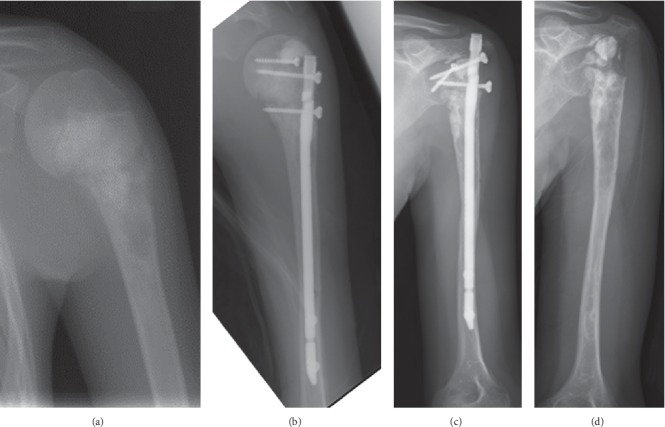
Case #6: 12-year-old female with osteosarcoma of the left proximal humerus. (a) Preoperative X-ray. (b) Immediately after treatment with osteoarticular extracorporeal irradiated autograft. (c) 14 years after operation, collapse of the graft and protrusion of the IM rod proceeded. (d) IM rod was removed.
